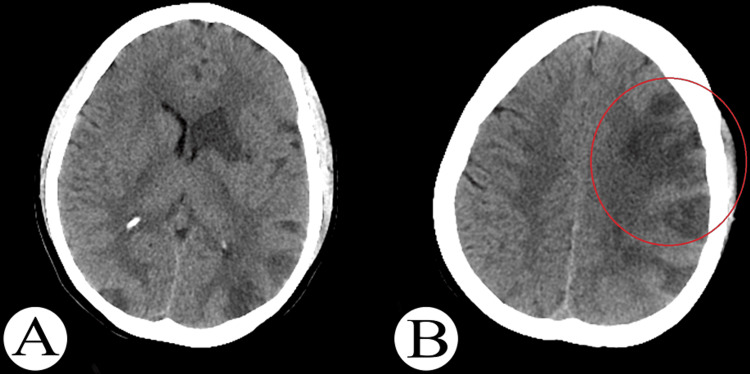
(A, B) Initial post-traumatic axial computed tomography of the brain showing multiple bilateral hemispheric hypodense area multiple strokes (red circle).
